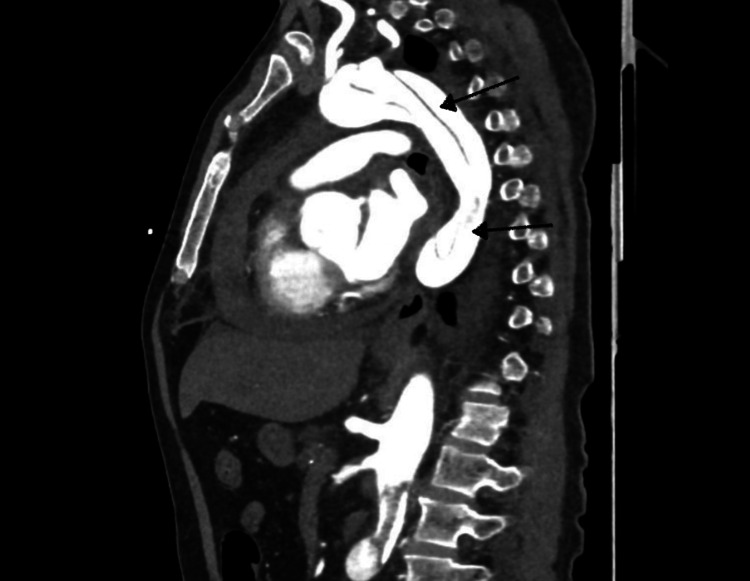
Sagittal view of CT aortogram showing Stanford type A AAD with the dissecting flap extending distally into the descending aorta.
